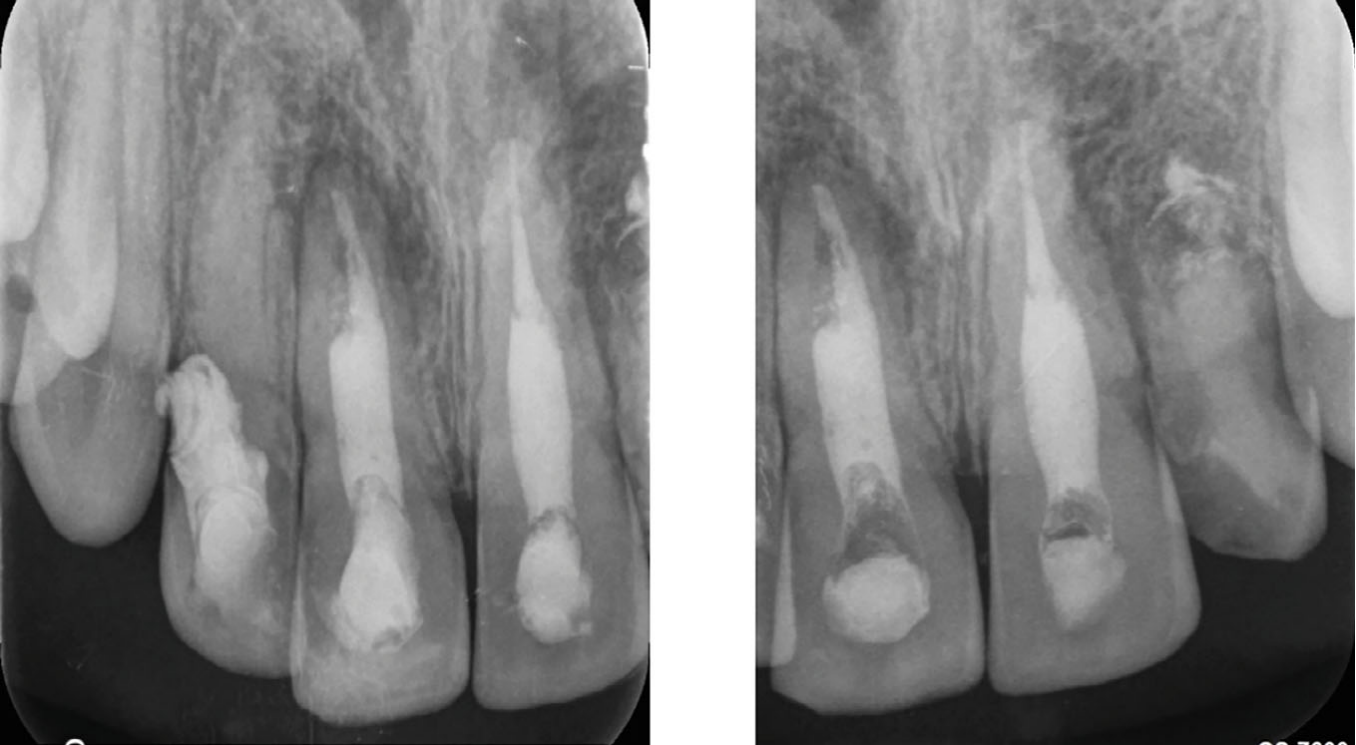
Intraoral periapical radiographs of 6 months' follow-up.
